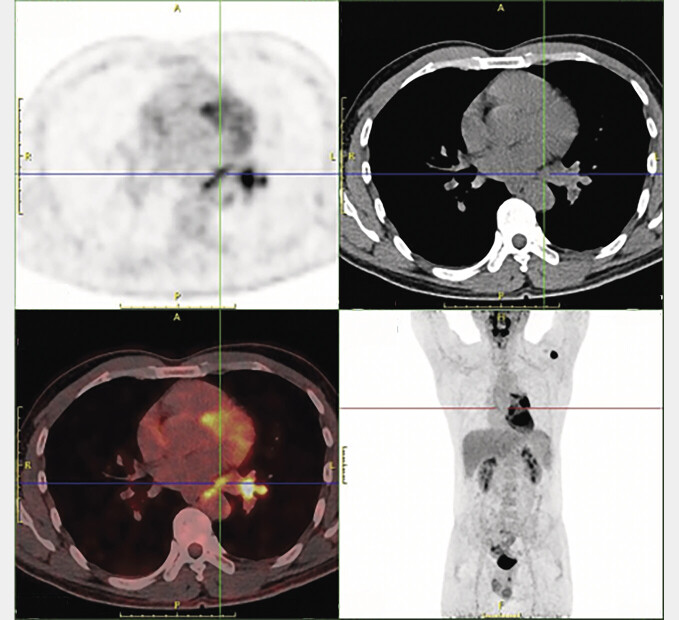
PET-CT detected a hypermetabolic lesion in the left inferior pulmonary vein.
